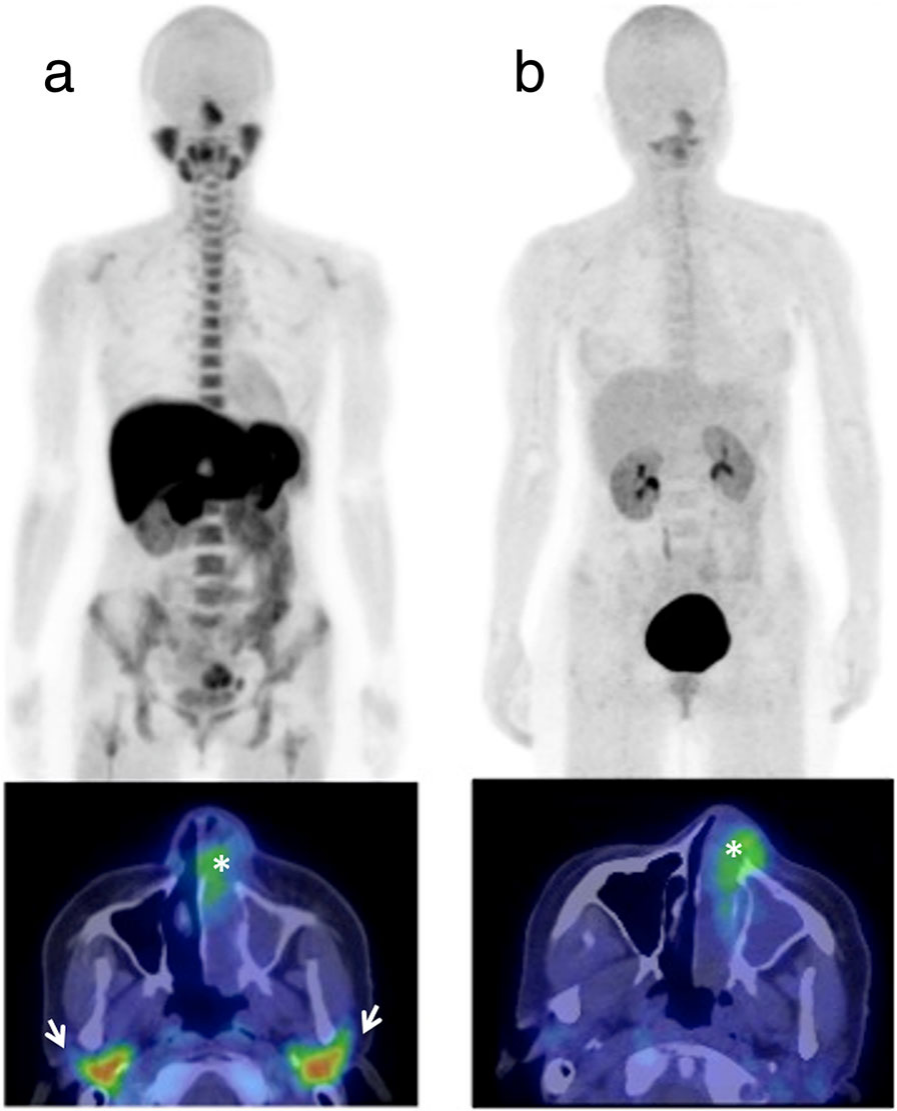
Representative ^11^C-Met and ^18^F-BPA PET/CT (patient No. 6). *Upper panel*: maximum intensity projection imaging. *Lower panel*: PET/CT fusion image. a
